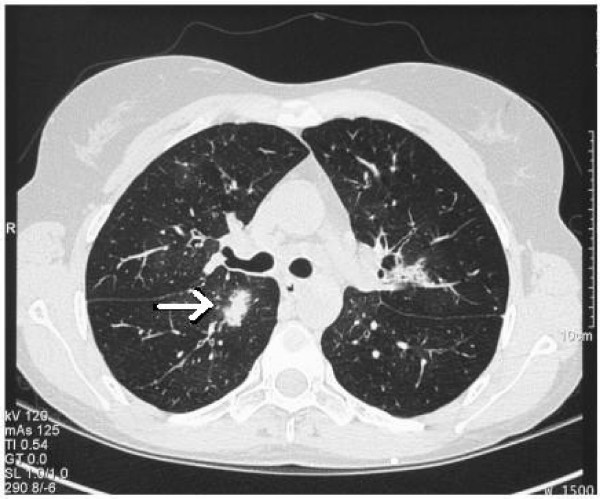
September 2005(CT Thorax): Halo sign. Note the so-called "halo sign", seen as a blush around the lesion. This sign is indicative of haemorrhage and highly suggestive of infection with an angioinvasive fungal organism.
